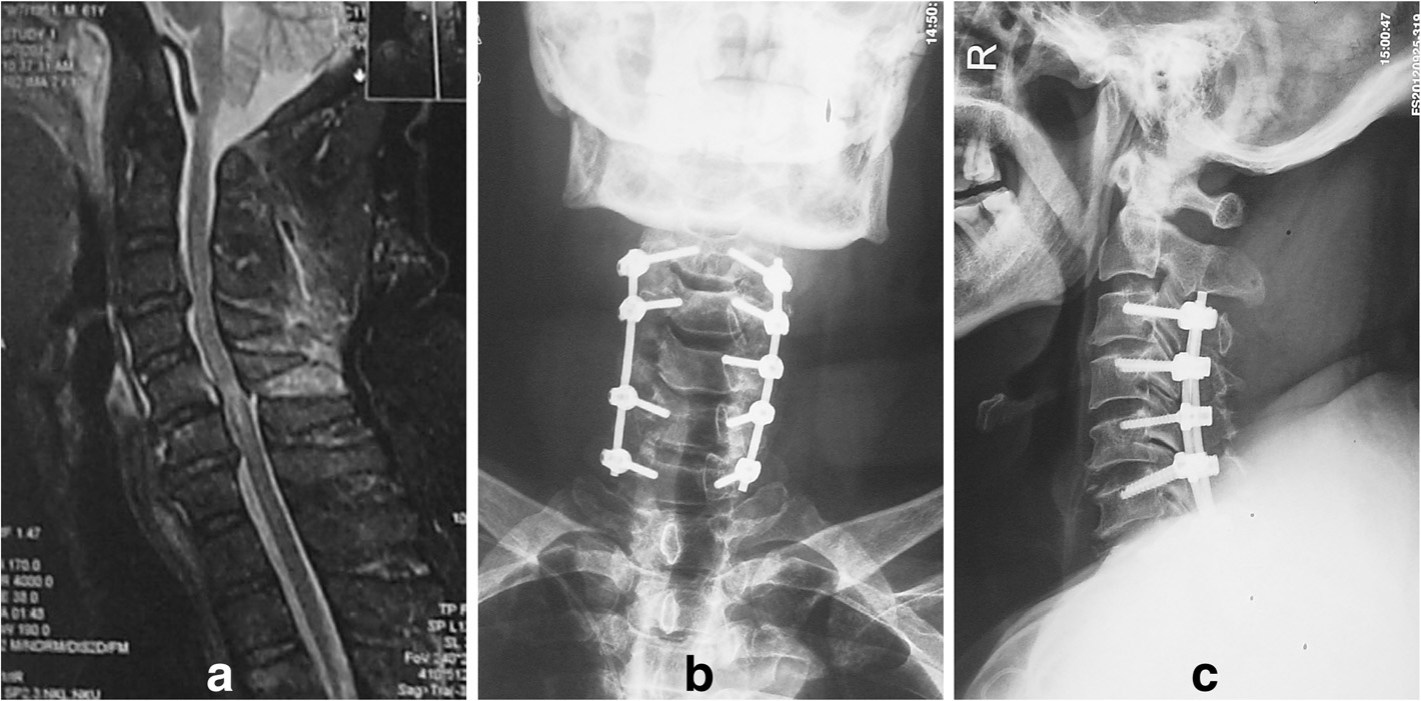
Radiologic studies of spinal stenosis and open-door posterior laminoplasty and transpedicle internal fixation. (a) Spinal stenosis between C3 and C4, C5 and C6, and C6 and C7 are shown with preoperative magnetic resonance imaging, in combination with posterior ligament complex injury. (b, c) Open-door posterior laminoplasty and transpedicle internal fixation at C3–C7.
